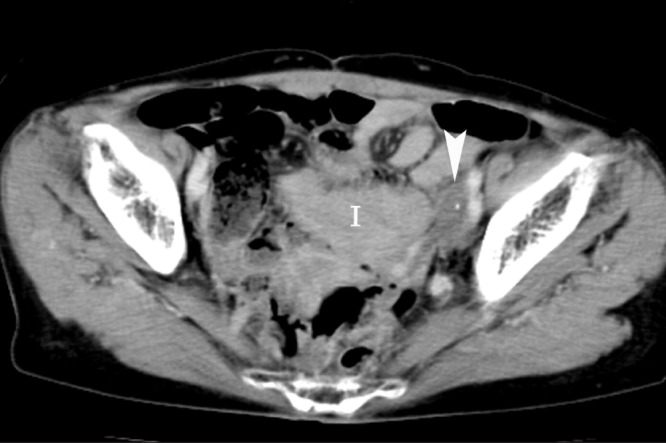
Post-contrast computed tomography performed 20 months after the initiation of radiotherapy showing a small area of low density with spotty calcifications (arrowhead) I = intestinal loops
